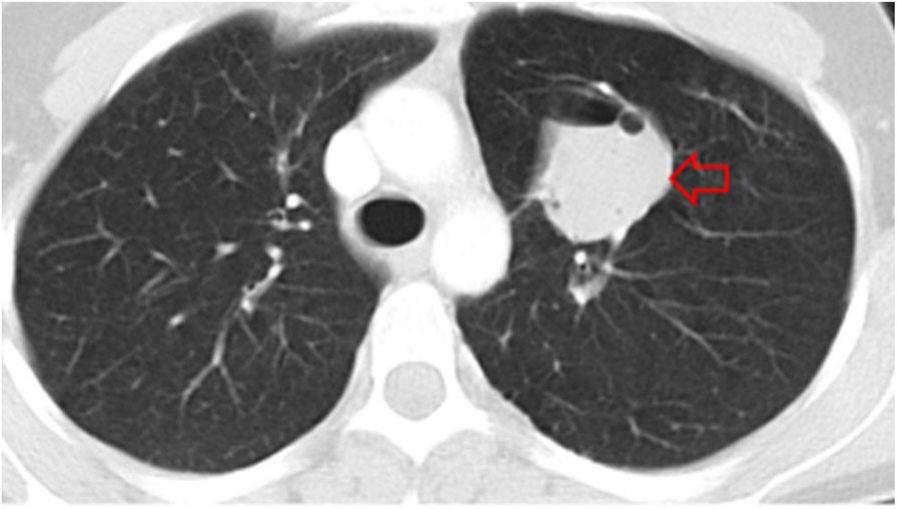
Axial CT image shows an opacification with smooth contours, which is a pathologically proven bronchogenic cyst (red arrow)
